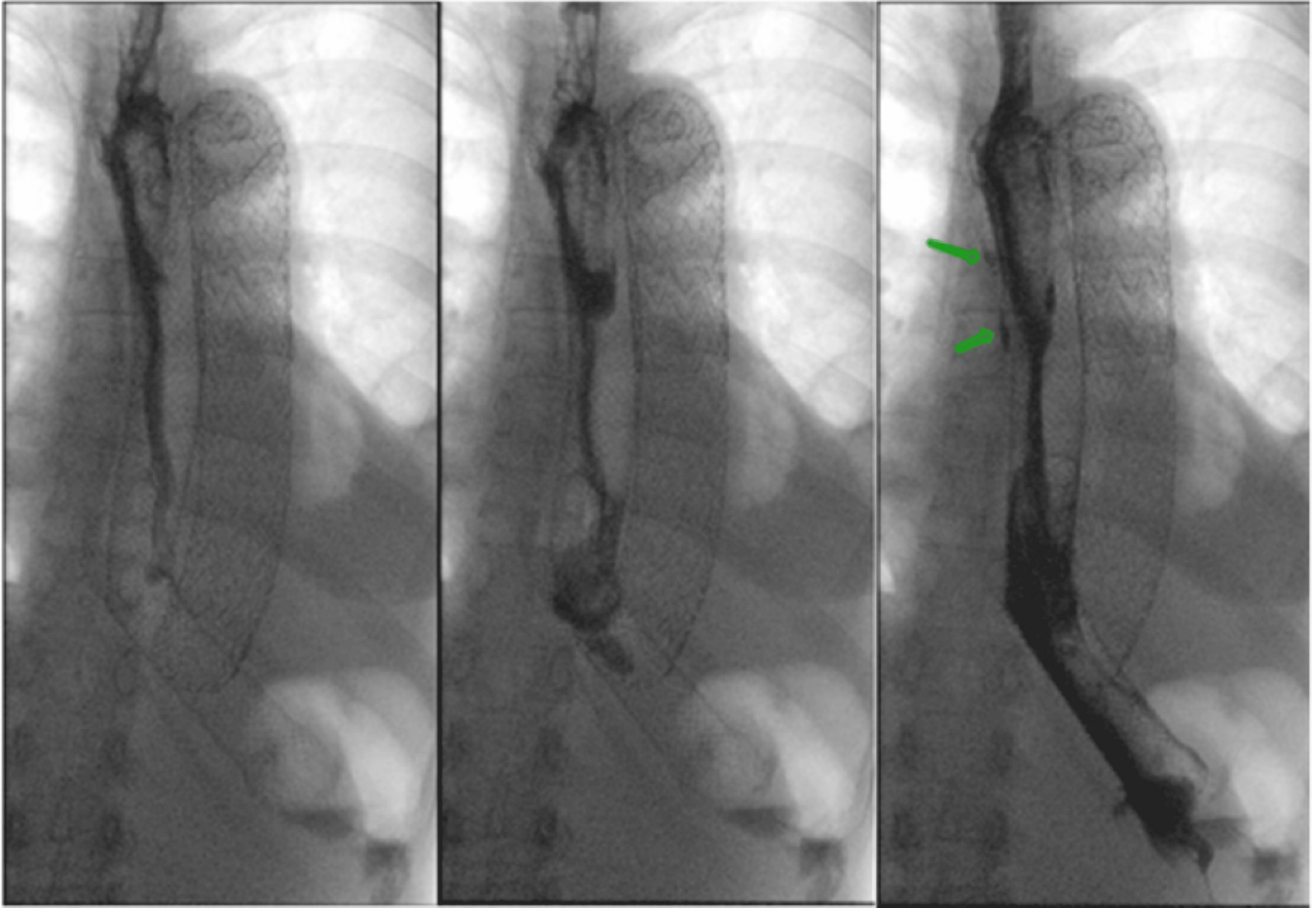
X-ray esophagus with oral contrast status post dual esophageal stent placement demonstrating continued pooling of contrast outside of the lumen of the stent
